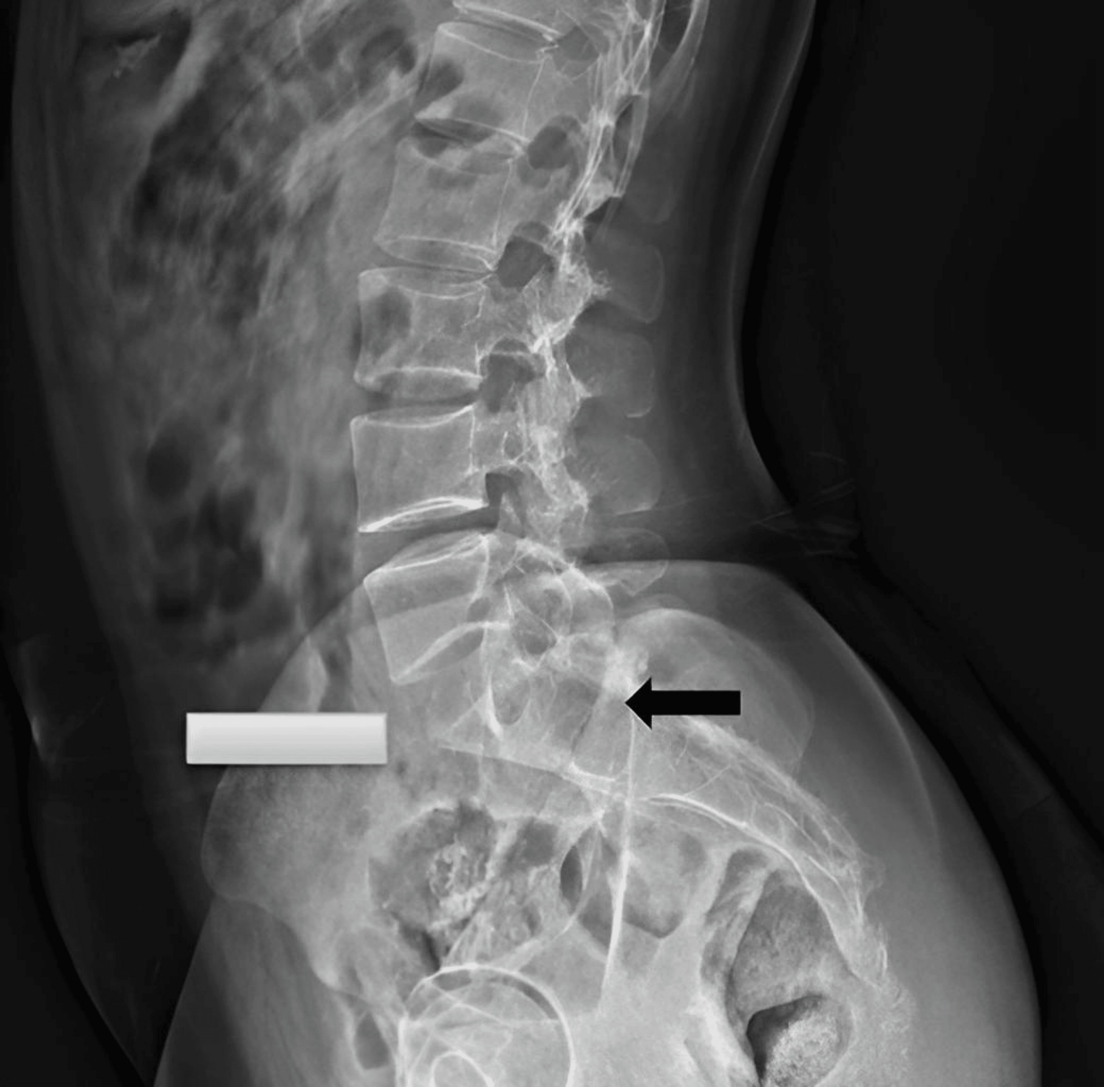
X-ray lumbar spine lateral view: An expansile, lytic lesion involving the S1 and S2 vertebral bodies (black arrow) demonstrated characteristic "soap-bubble" radiolucency with cortical thinning.
